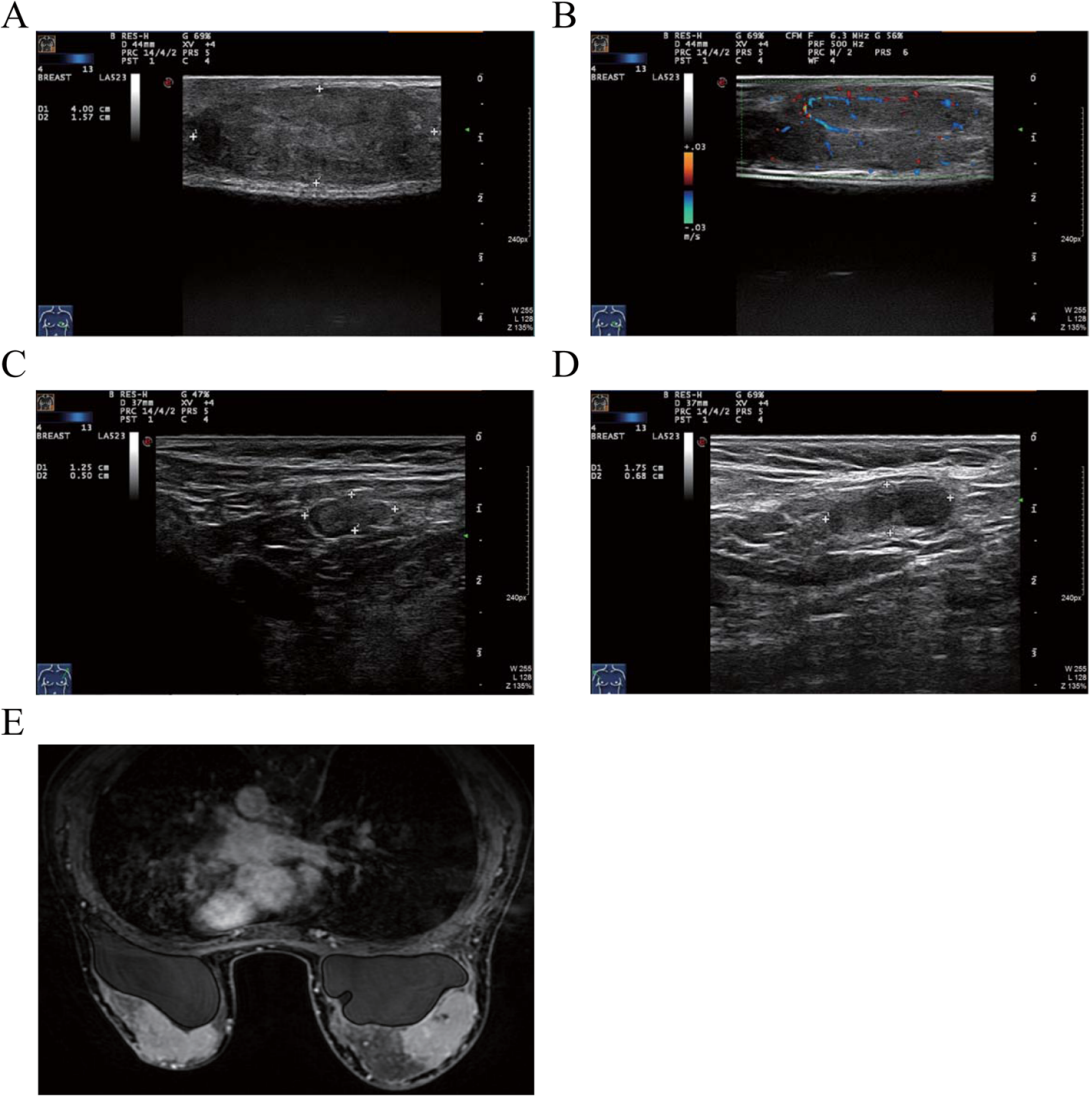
The ultrasound and MRI results of the breast masses. A Breast ultrasonography revealed masses with well-defined hypoechoic lesions in the left breast. B Masses with well-defined hypoechoic lesions were found in the right breast. C Hypoechoic nodules were seen in the left axillae (1.25 cm × 0.50 cm). D Hypoechoic nodules were seen in the right axillae (1.75 cm × 0.68 cm). E MRI showed hypointensity on T1-weighted images and hyperintensity on T2-weighted images
